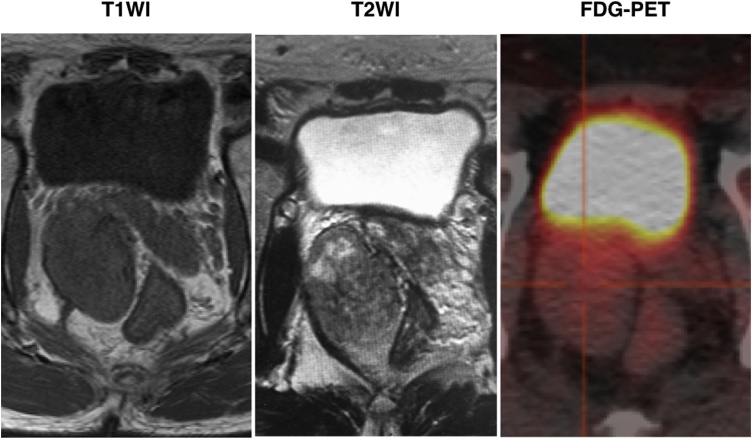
Preoperative MRI and FDG-PET findings of Case 1. Preoperative MRI showed a well-demarcated T1 low, T2 mixed intensity extramural tumor behind the seminal vesicle that was located between the right lateral wall of the low rectum and the right lateral pelvic wall. In FDG-PET, an accumulation of SUV was not shown corresponding to a 5.3 × 3.6 cm mass was identified.
